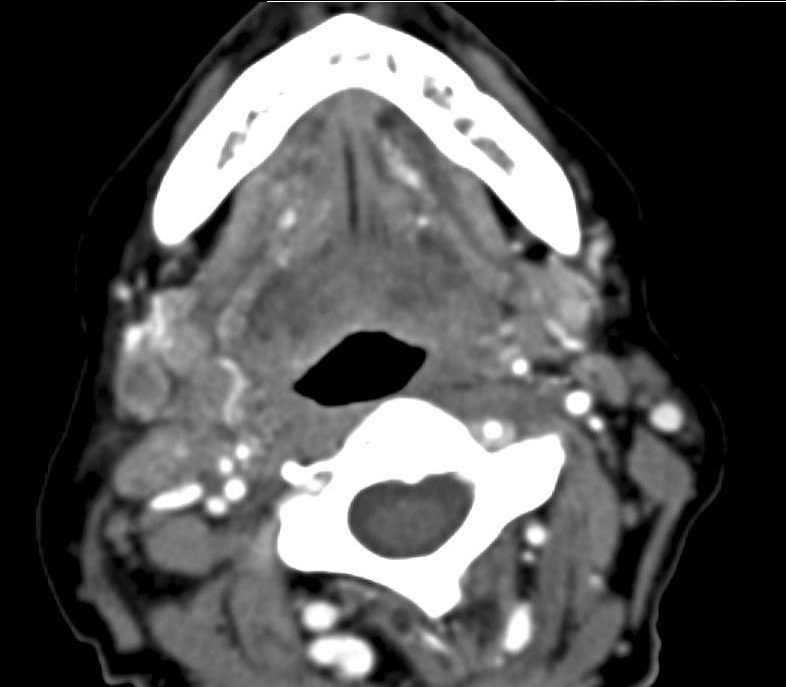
Local recurrence of the maxilla first detected by CT-scans.
